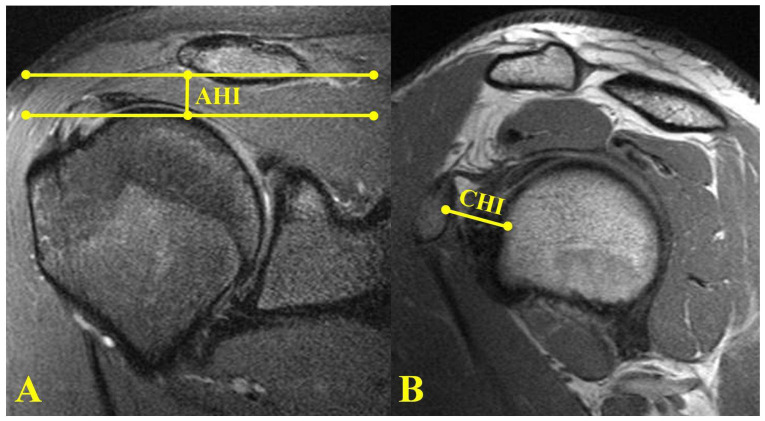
(A) Coronal MRI of shoulder showing measurement of coronal acromiohumeral interval (AHI). (B) Sagittal MRI of shoulder showing coracohumeral interval (CHI).
